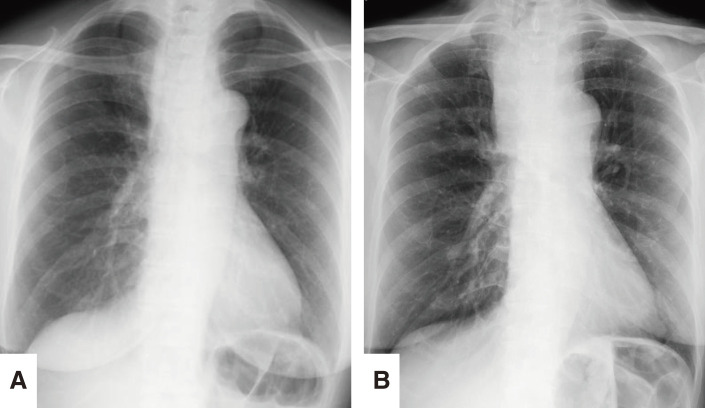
(A) A chest radiograph immediately after the EUS-FNA showing a normal mediastinum. (B) A chest radiograph 3 days after the EUS-FNA showing recurrent mediastinal widening. EUS-FNA, endoscopic ultrasound-guided fine-needle aspiration
